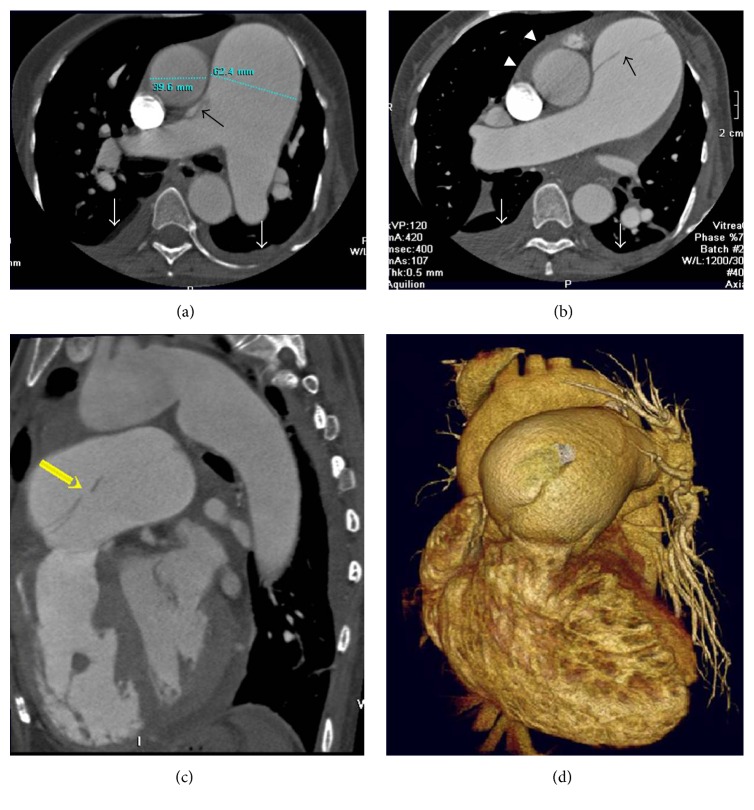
Intimal flap features determined on CT angiography. Transverse sections of CT pulmonary angiography (a, b) showing dilated central pulmonary arteries with an intimal flap (black arrow) in the main pulmonary trunk and right pulmonary artery. Pericardial effusion (white arrowheads) and bilateral pleural effusion (white arrows) are also noted. Sagittal MPR reconstruction (c) revealed the primary entry tear (arrow) in the proximal main pulmonary trunk and retrograde extension of the intimal flap to the pulmonary valve. Volume rendered images (d) vividly demonstrated the relationship between the intimal flap and pulmonary artery root.
